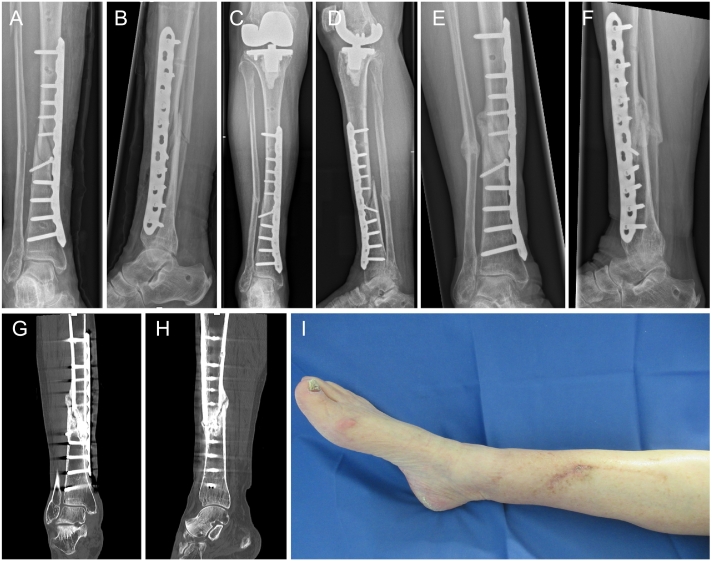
Anterior-posterior (Ap) (A) and lateral (B) X-ray after open reduction, plate and lag screw fixation of the distal tibia shaft fracture. Ap (C) and lateral (D) X-rays at 6 weeks with first signs of callus formation. Ap (E) and lateral (F) X-rays at 5 months with bridging callus formation and full consolidation of the tibia and of the fibula fracture confirmed by representative coronal (G) and sagittal (H) CT sections. Clinical image of the distal leg (I) showing undisturbed wound and soft tissue healing at 5 months without any signs of argyria (blue-grey decolorization of the skin around the silver-coated plate) or other silver-specific side effects. (For interpretation of the references to colour in this figure legend, the reader is referred to the web version of this article.)
